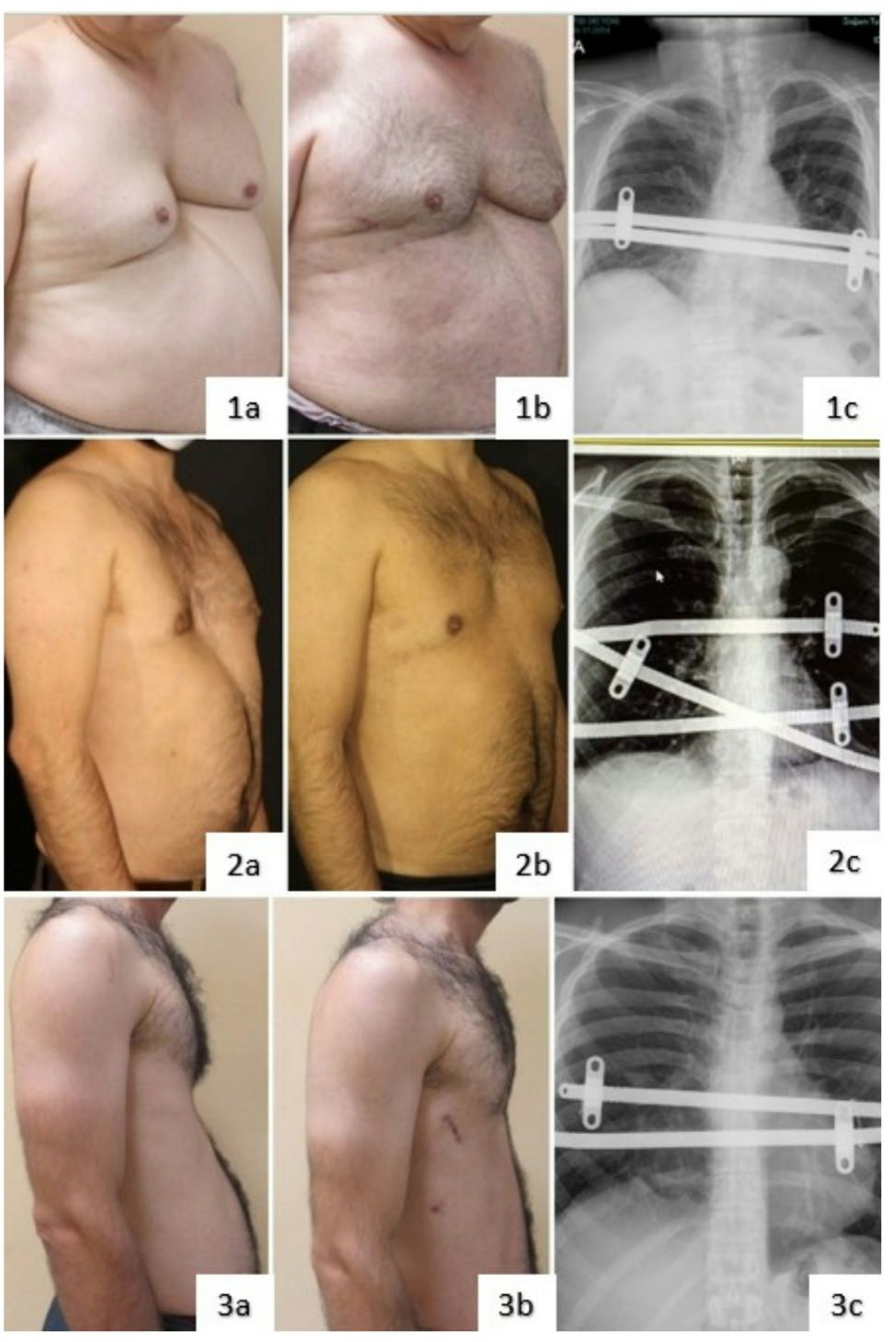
Representative preoperative and postoperative clinical photographs and chest radiographs of adult patients aged over 40 years who underwent minimally invasive repair of pectus excavatum using the Nuss procedure (1a–1c) A patient treated with two parallel bars (2a–2c) A patient treated with three bars using a cross-bar configuration (3a–3c) A patient with deep asymmetric pectus excavatum treated with two parallel bars
